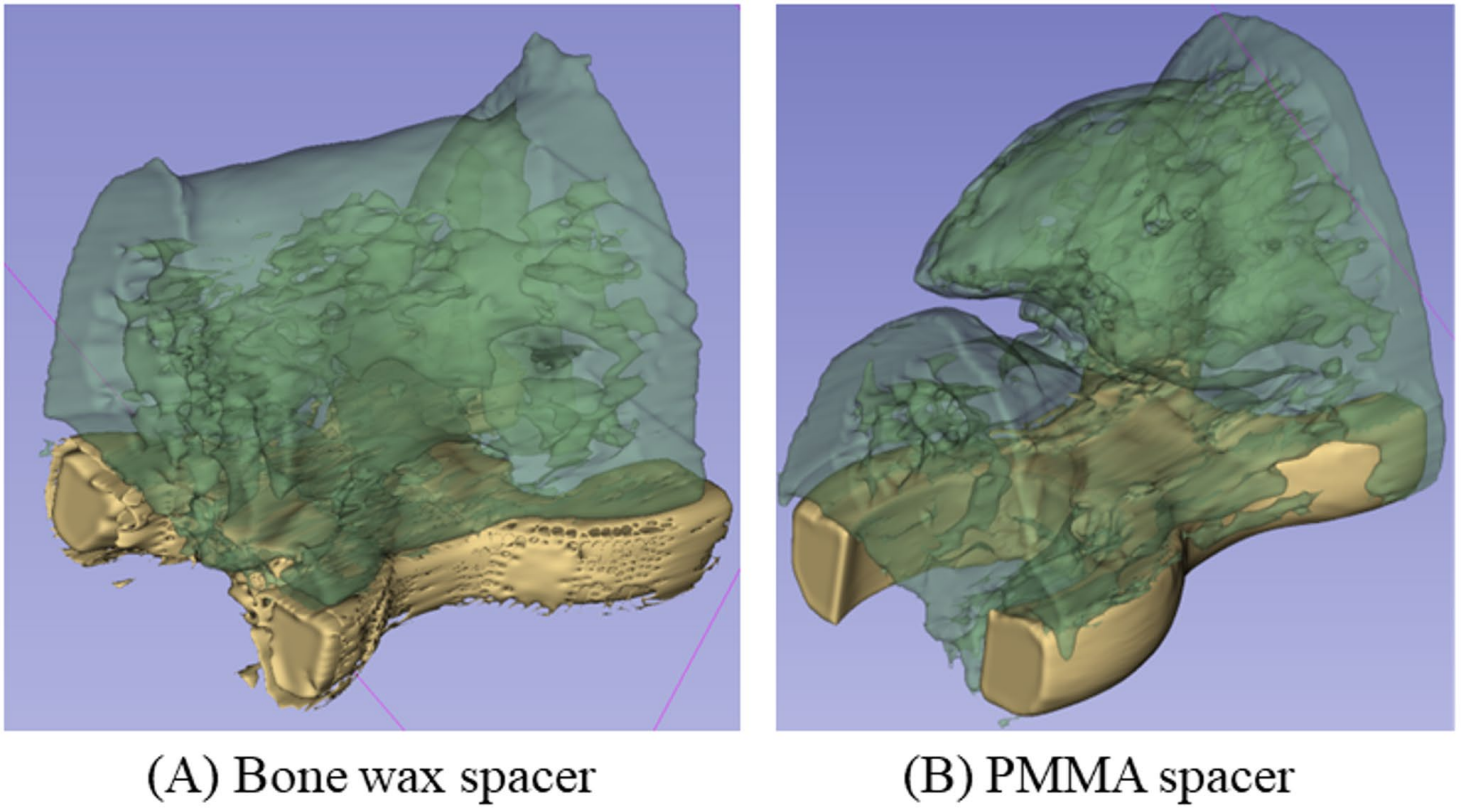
CT evaluation of bone formation in the explanted bone. This 3D image shows the segmentation of the bone defect only. In the bone wax spacer, the allograft bone is fully cross-linked, the edges of the allograft bone become cortical bone, the center is trabecular bone, and the allograft bone remodels to form a medullary cavity. Osteogenesis of the allograft bone is also observed in the PMMA spacer, but not to the point of complete cross-linking formation
